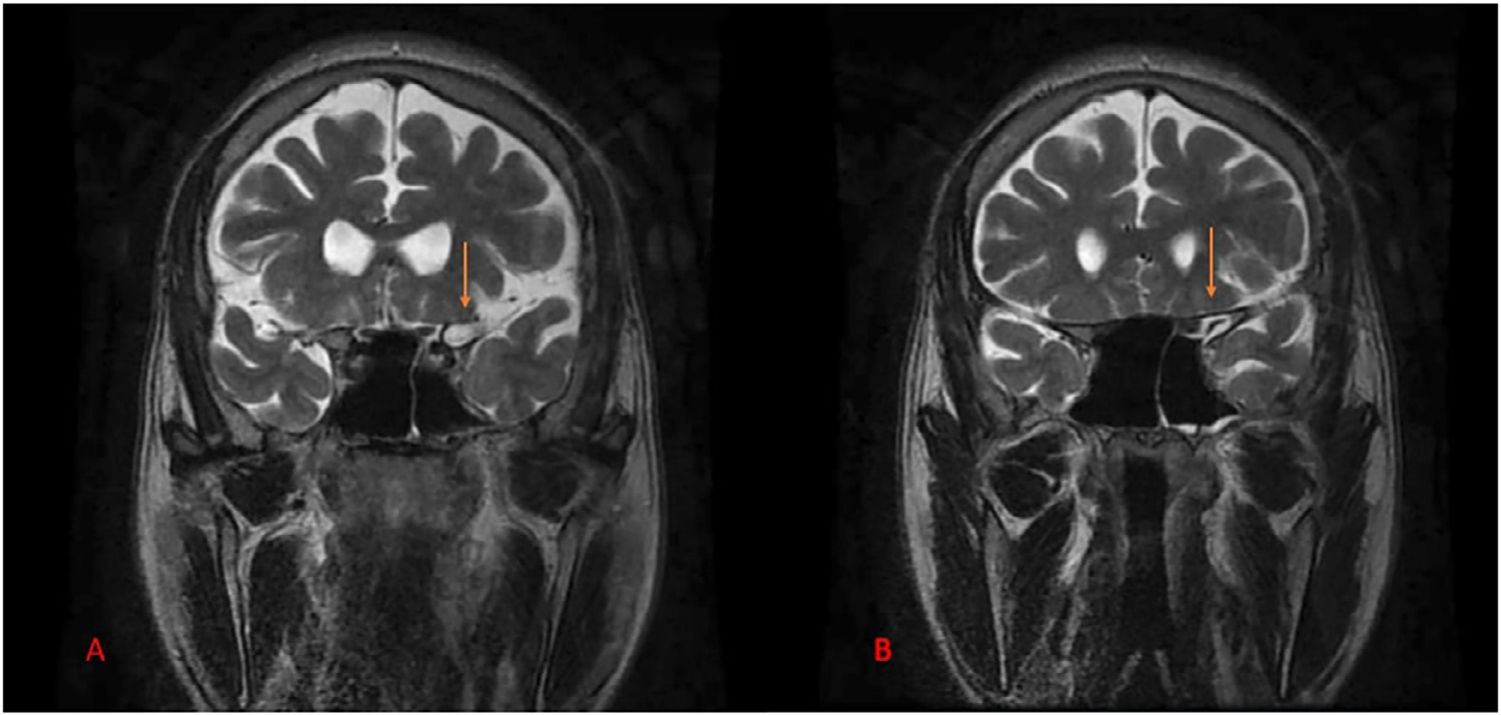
MRI brain coronal image T2 weighted imaging (A) and (B) showing an intra-diploic lesion measuring approximately 14 × 7 mm adjacent to the left cavernous sinus, which appears isointense to CSF and is noted communicating with the greater wing of the left sphenoid bone.
